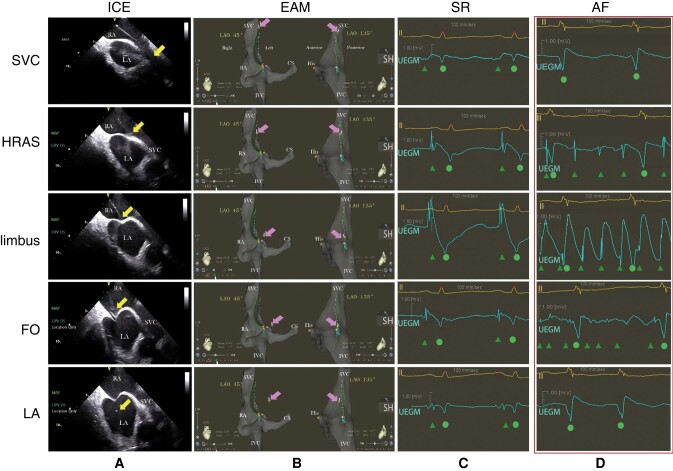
The changes on the UEGM tip during TSP with ICE verification. The entire assembly was pulled down from the SVC to the FO. The needle tip location was confirmed by intracardiac echo (*A*, indicated by the yellow arrows) and visualized by 3D-EAM using left anterior oblique 135° and 45° projections (*B*, indicated by the pink arrows) at various atrial sites, including the SVC, the high right atrial septum, the muscular limbus, the FO, and the LA chamber. Simultaneously, the morphology and amplitude of the unipolar electrogram derived from the needle tip were recorded (*C* for SR and *D* for another case with AF). AF, atrial fibrillation; CS, coronary sinus; EAM, electroanatomic mapping; FO, foramen ovalis; His, His bundle; HRAS, high right atrial septum; ICE, intracardiac echocardiography; IVC, inferior vena cava; Limbus, the muscular limbus of the foramen ovalis; LA, left atrium; RA, right atrium; SR, sinus rhythm; SVC, superior vena cava; (UEGM) tip, (a unipolar electrogram) derived from a transseptal needle tip. Green triangle indicates unipolar atrial potentials recorded from the needle tip. Green circle denotes far-field unipolar ventricular potentials.
